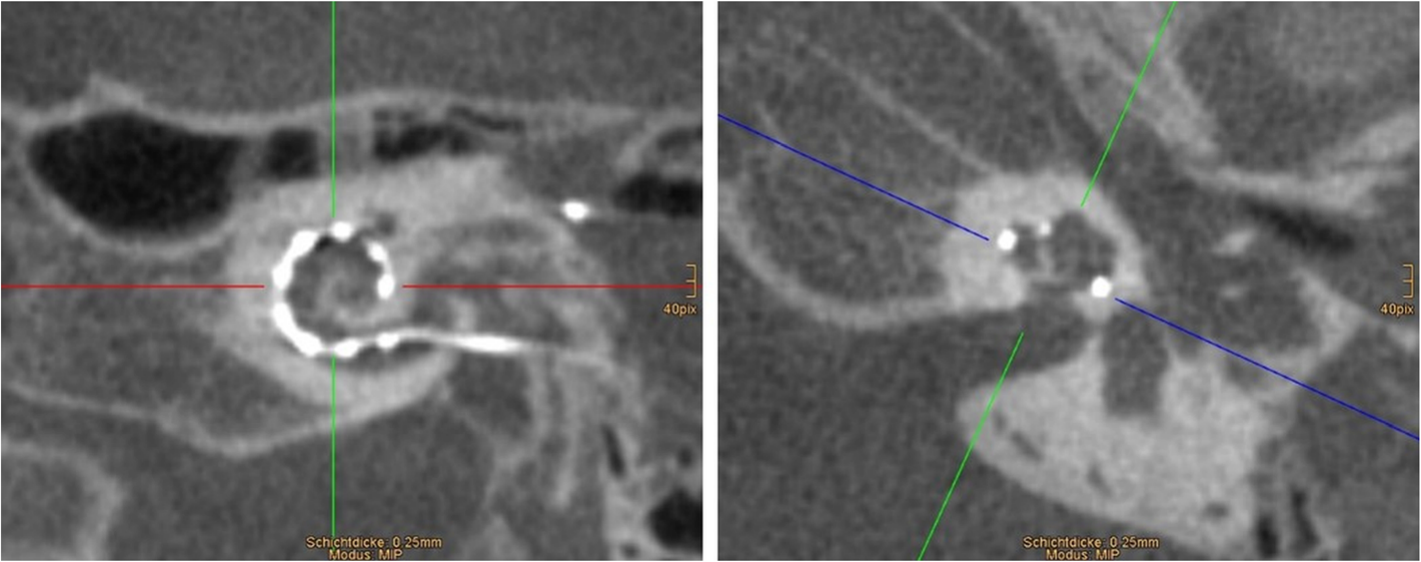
Postoperative evaluation of cochlear implant location using multiplanar mid-modiolar reconstructions. CBCT paracoronal reconstruction at the basal turn (left image) and paraaxial reconstruction through the modiolus (right image). Maximum intensity projections may be used to visualize the entire electrode array
